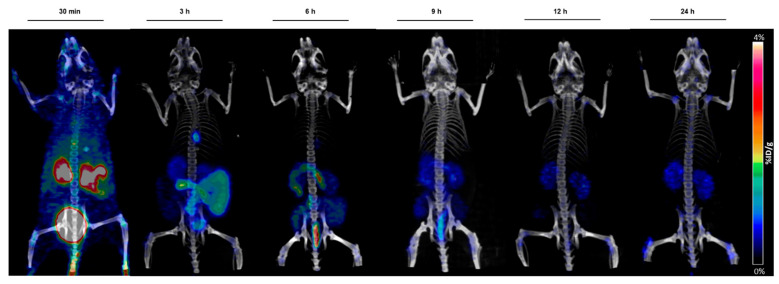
Maximum image projections (MIPs) obtained from static PET/CT scans at different time points. A hepatobiliary and renal excretion is clearly visible as well as a low accumulation in bones and kidneys 24 h p.i. Scale bar: 0–4%ID/g.
